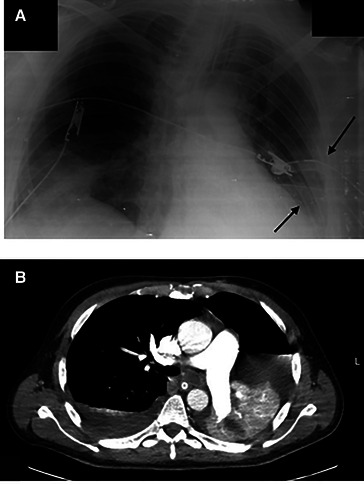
(A) Portable x-ray demonstrating two chest tubes inserted at the left side (arrows). (B) CT scan showing Collapsed left lung, causing ipsilateral deviation of the mediastinum and trachea, mild to moderate left sided pneumothorax, and mild bilateral pleural effusion. Focal area of consolidation seen at the right lower lobe with surrounding ground glass opacities.
